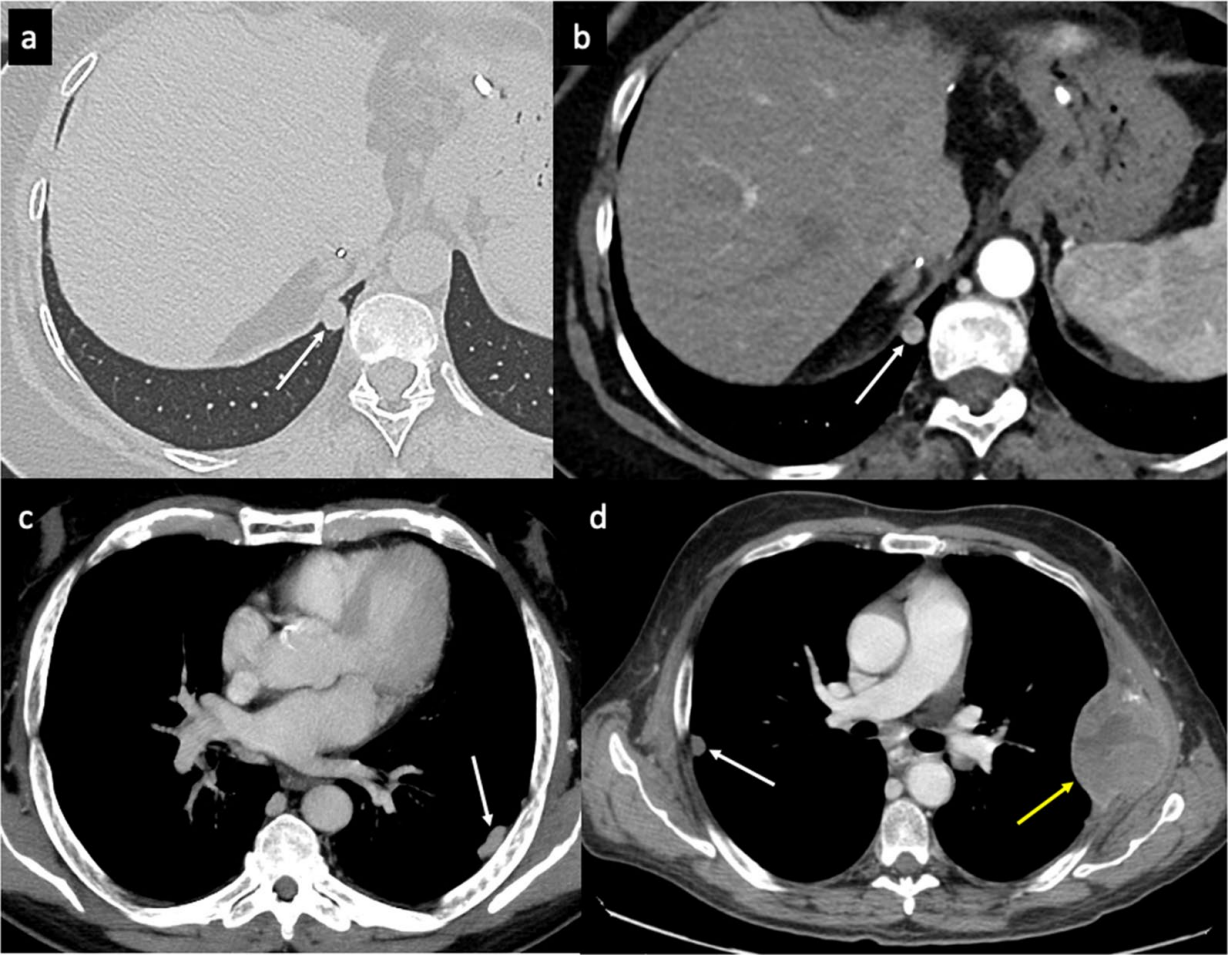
Lung and pleural recurrence. Axial unenhanced (a) and contrast-enhanced CT images on the arterial phase (b) exhibit a small lung recurrence in the right lower lobe (arrows). Notice the presence of hyperenhancement on the arterial phase due to the hypervascularity of the primary tumor. Axial contrast-enhanced CT images (c, d) show different pleural recurrences (white arrows) with the appearance of plaques or nodules. Notice the coexistence of rib metastasis (yellow arrow) with bone erosion
